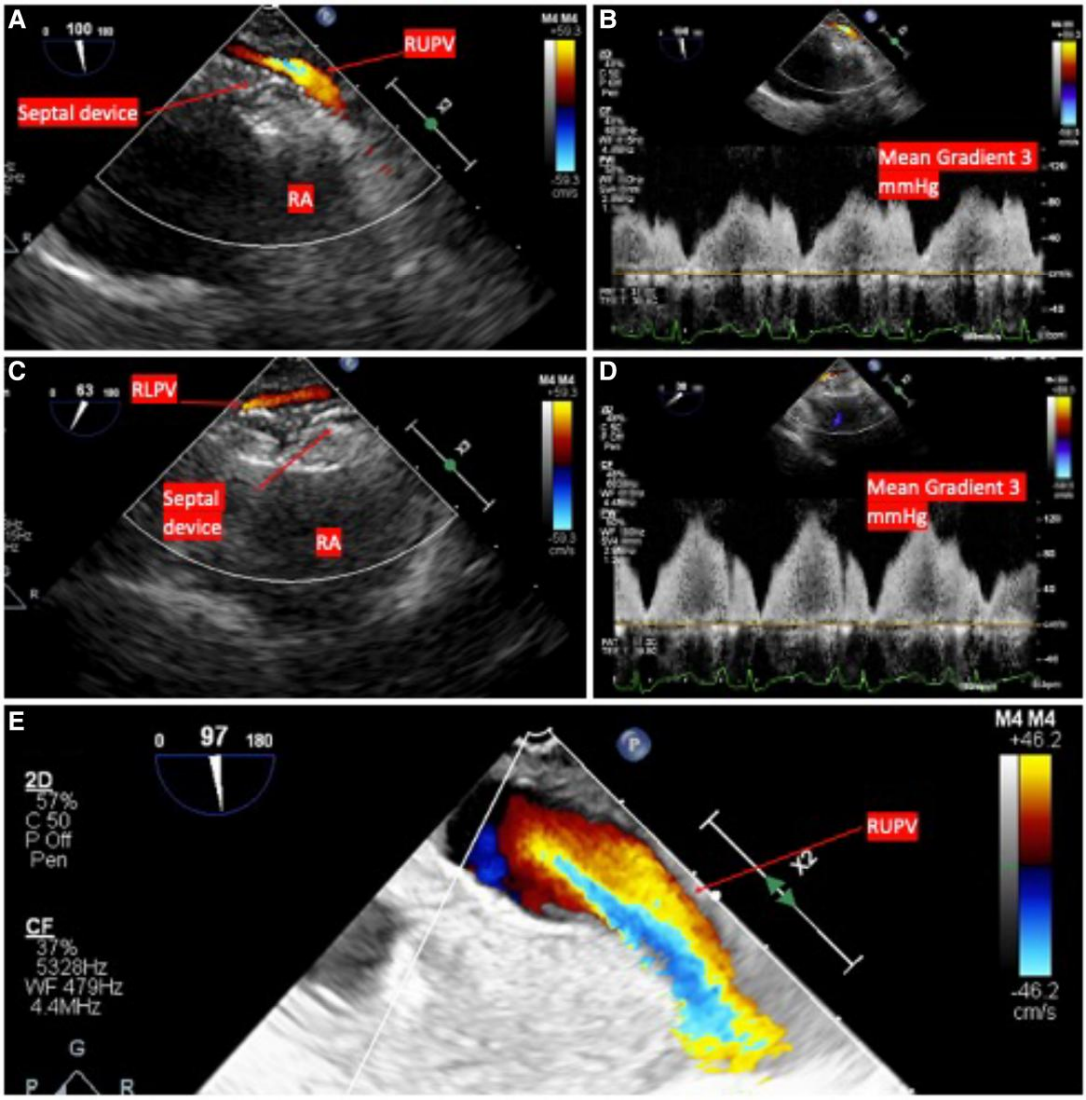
Bicaval view on transthoracic echocardiography. (*A* and *B*) The right upper pulmonary vein and (*C* and *D*) the right lower pulmonary vein, both with colour Doppler and continuous wave Doppler with a mean gradient of 3 mmHg with the septal occlusion device in place. (*E*) The right upper pulmonary vein with improvement in size and flow after the device was removed. RA, right atrium; RUPV, right upper pulmonary vein; RLPV, right lower pulmonary vein.
